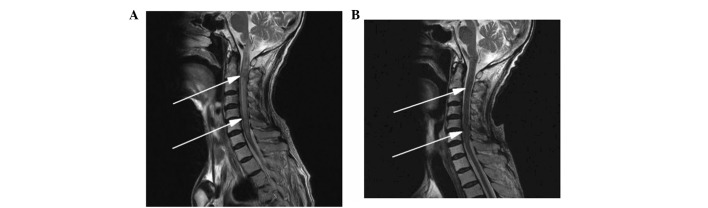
Comparison between the MR images of the cervical spinal cord prior to and following chemotherapy. Sagittal T2WI (A) prior to chemotherapy (MR 10-28-2009) showing increased T2WI signals and abnormal morphological augmentation in the medulla oblongata and cervical cord indicating metastatic lesions (indicated by the two arrows), and (B) following chemotherapy (MR 11-23-2009) showing decreased T2WI signals in the medulla oblongata and cervical cord consistent with the signals of normal tissues, and marked improvement in the abnormal morphology (as indicated by the two arrows). MR, magnetic resonance; T2WI, T2-weighted image.
